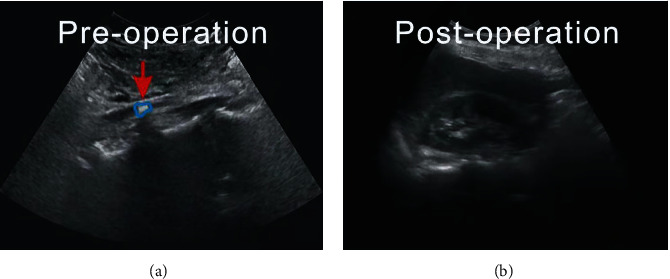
Ultrasound scan image of ureteral stones in subject 2 ((a) original scan image and (b) postoperative scan image). The arrows in the medical images point to the ureteral stones, which show up clearly after image enhancement.
